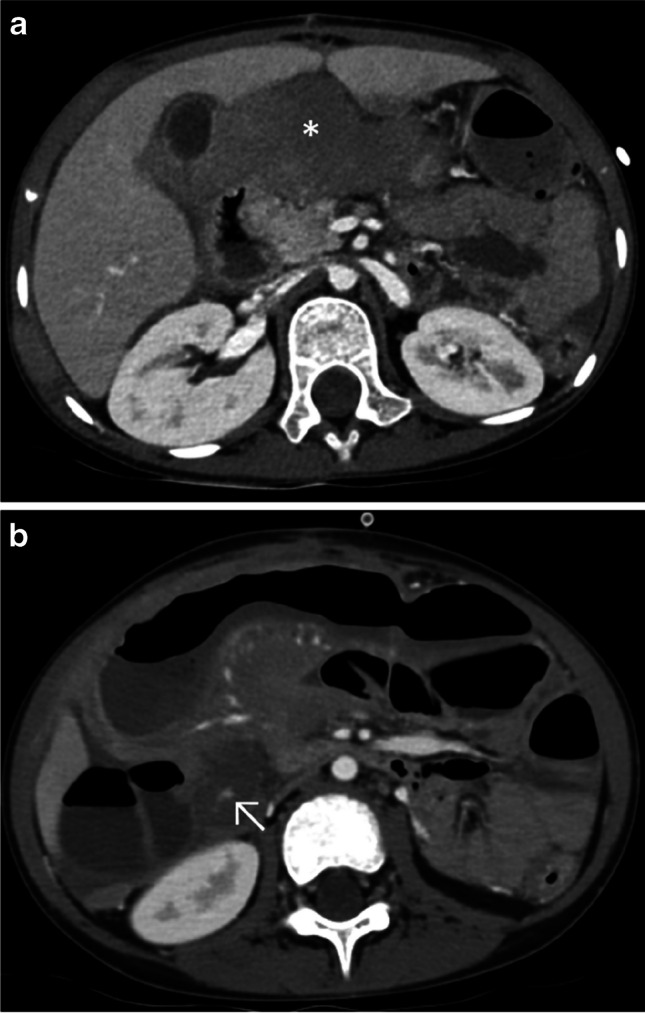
A 7-year-old boy with a history of a rapidly progressive Burkitt lymphoma. Axial computed tomography, post-contrast, following a split-bolus protocol, upper-abdominal images (soft tissue window). a Tumoral mass seen as a hypo- to isodense space-occupying lesion (*asterisk*). b Oncological complication with active intestinal hemorrhage, seen as hyperdense foci indicating extravasation of contrast media (*arrow*)
